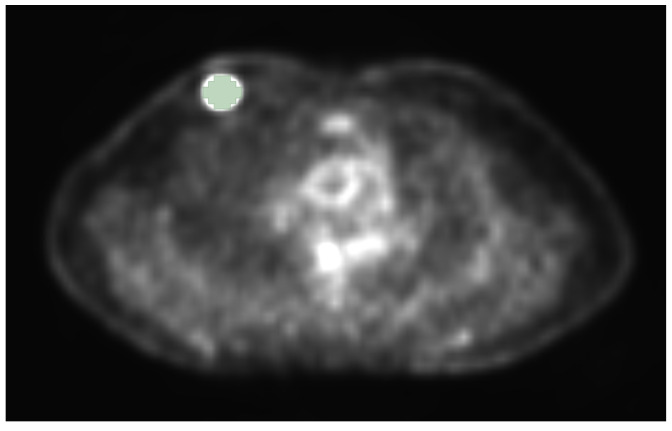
The 3D slicer was used to segment the primary lesions of IDC patients with cN0, and the threshold was SUV = 2.5.
